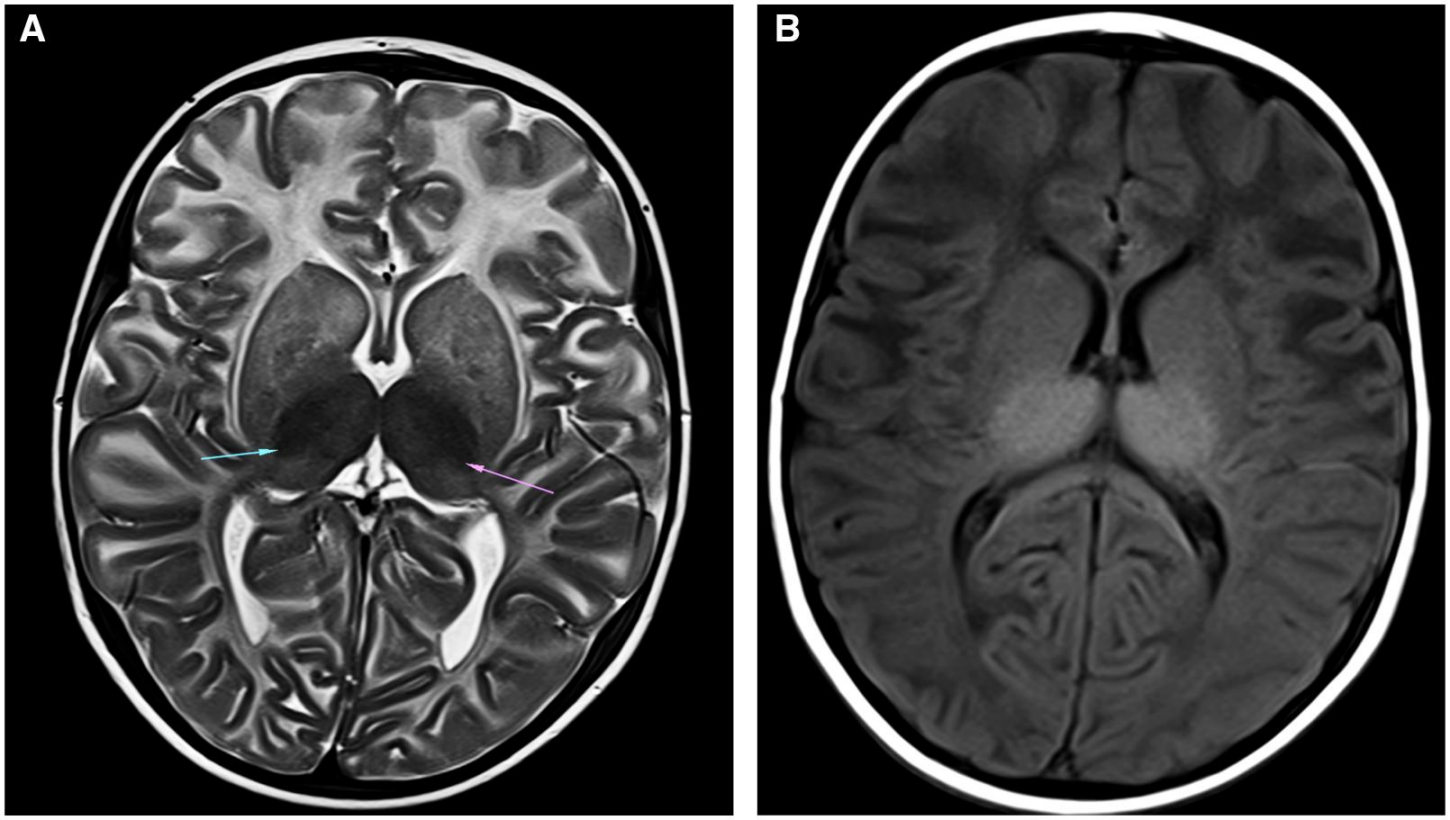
(A) Axial T2-weighted image showing bilateral bulky and hypointense thalami (arrows). (B) Axial T1-weighted image showing bilateral bulky and hyperintense thalami.
